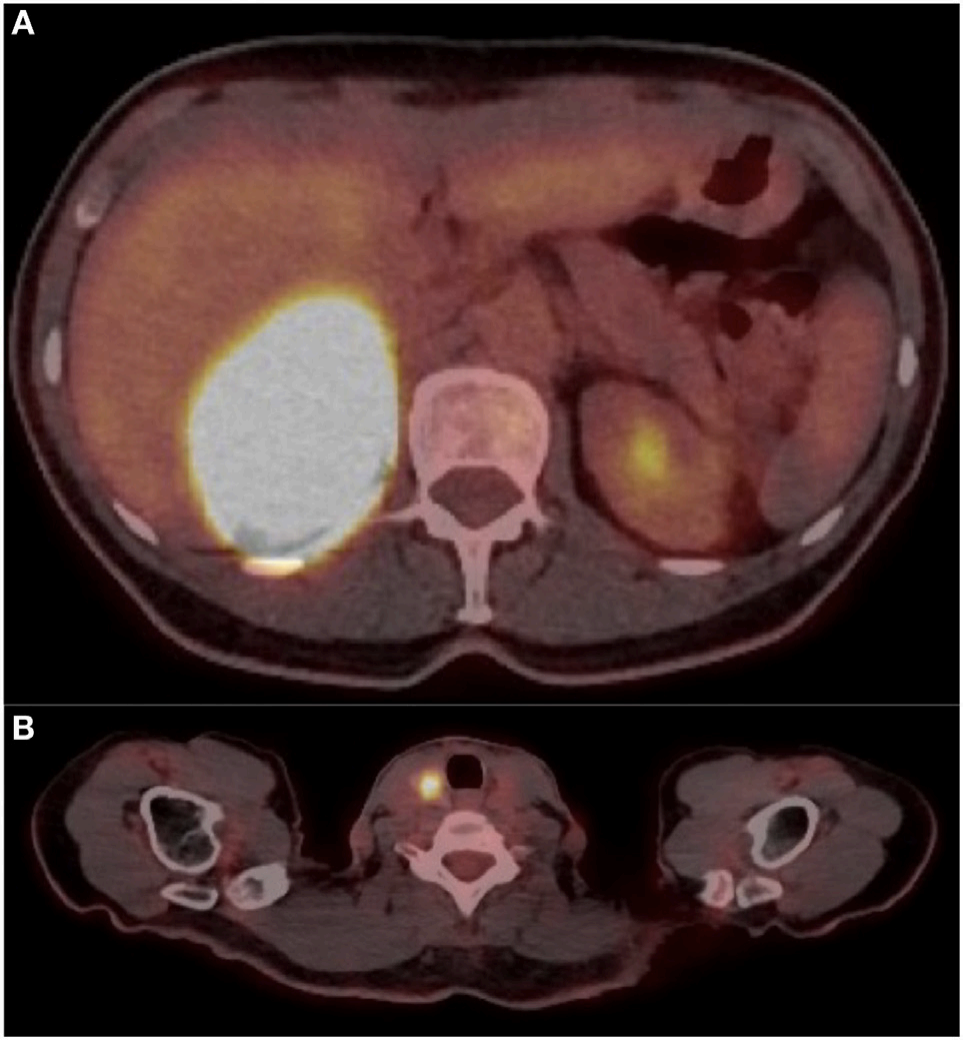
(A) Cross-sectional image on FDG positron emission tomography (PET) showing right adrenal mass at SUV max 88.7. (B) Cross-sectional image on FDG PET showing right thyroid mass at SUV max 10.6.
